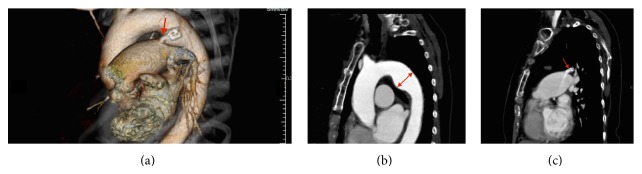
(a) A volume rendering CT image showing the PDA (red arrow). (b) A curved planar CT reformation image showing the TAA (red arrow; diameter, 41 mm). (c) A curved planar CT reformation image showing the PDA (red arrow; diameter, 6 mm) with contrast enhancement and calcification.
